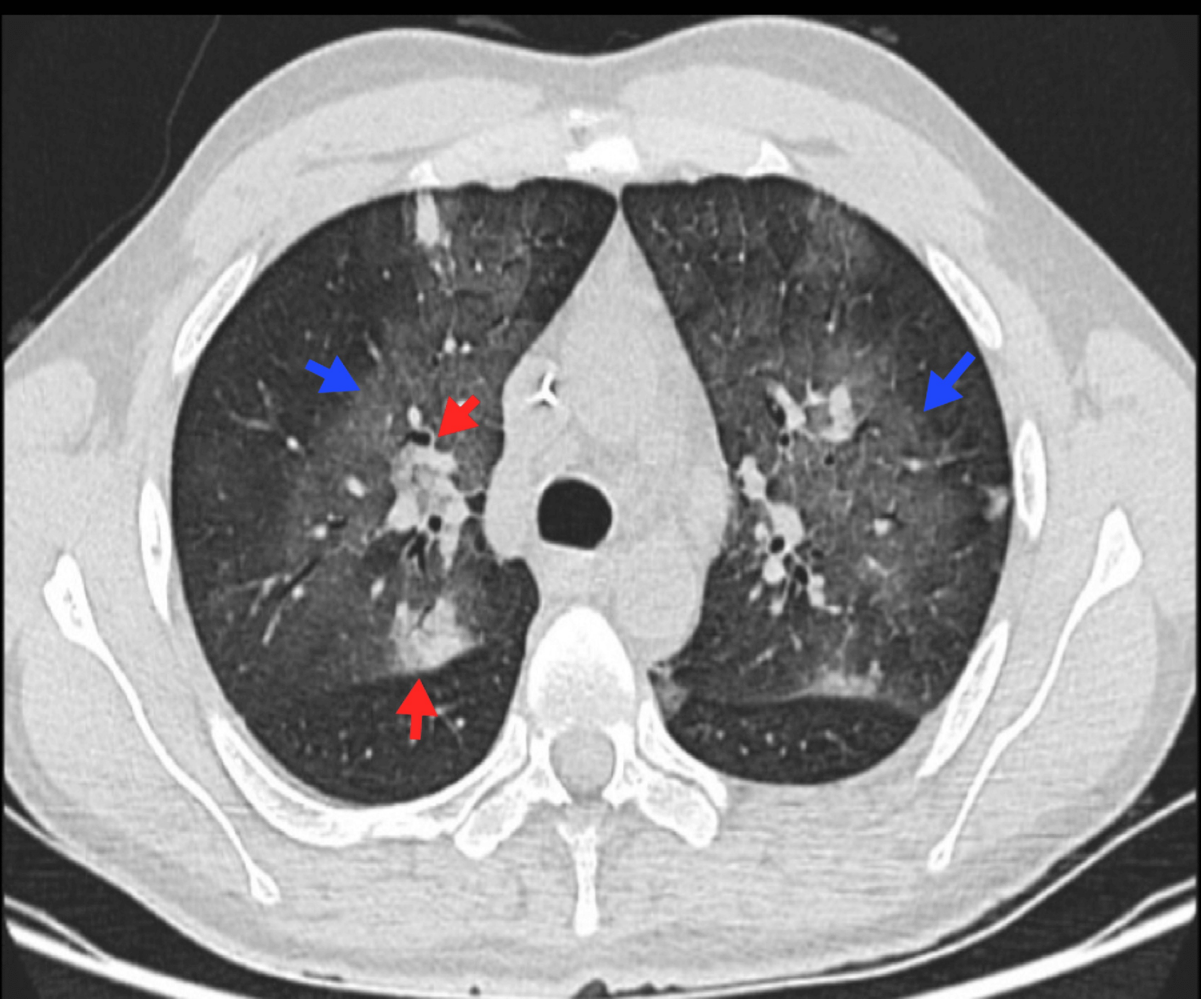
Differentiation syndrome and opportunistic mycosis A 22-year-old male patient with acute promyelocytic leukemia developed dyspnea after 10 days of ATRA therapy. Chest CT revealed central ground-glass opacities in the lung parenchyma, predominantly in the upper and middle lobes (blue arrows), raising suspicion for differentiation syndrome. In addition, pulmonary nodules and peribronchovascular consolidations were observed (red arrows), and galactomannan antigen was positive in the BAL. Treatment for suspected aspergillosis with voriconazole was initiated, resulting in clinical improvement. ATRA, all-trans retinoic acid; BAL, bronchoalveolar lavage Image courtesy: Pulmonology Service of the Internal Medicine Department, University of Antioquia. Consent for open access publication was obtained from the patient.
